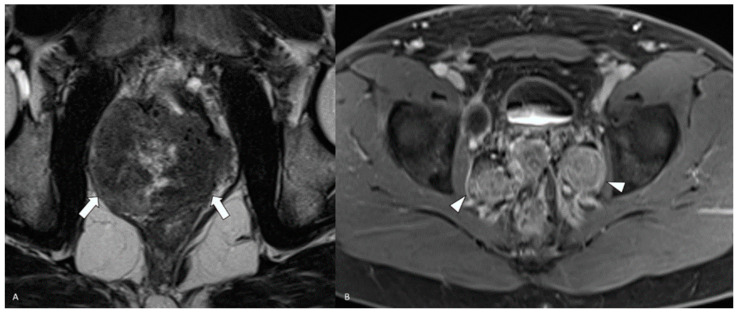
MRI of the pelvis: (A) axial T2 demonstrates a large heterogeneously hypointense lesion in the prostatic bed (white arrows). (B) Axial contrast-enhanced T1 fat-saturated sequence shows bilateral pelvic adenopathy (white arrowheads).
